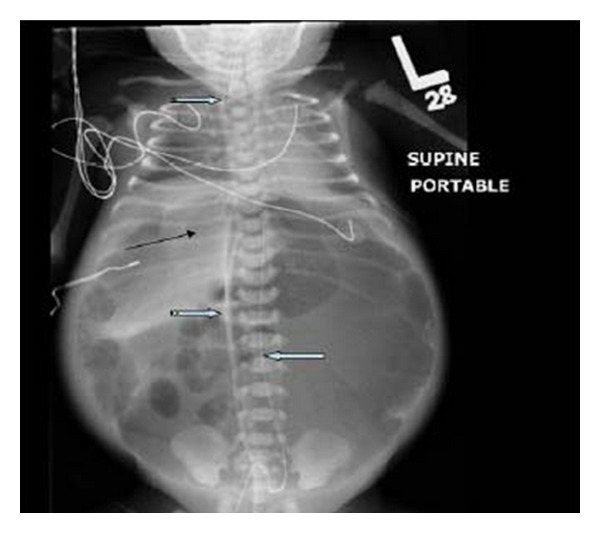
A baby gram X-ray showing a severely distended abdomen with free intraperitoneal air in front of liver (black arrow) and umbilical artery catheter (right striped block arrow) and umbilical vein catheter (left block arrow) in situ. An endotracheal tube (notched block arrow) is noted in situ and both diaphragms are significantly elevated.
